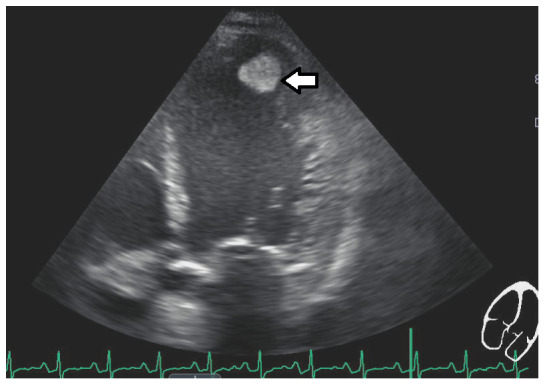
Echocardiogram (Apical 2 chamber view) showing dilated left ventricle showing apical thrombus.
